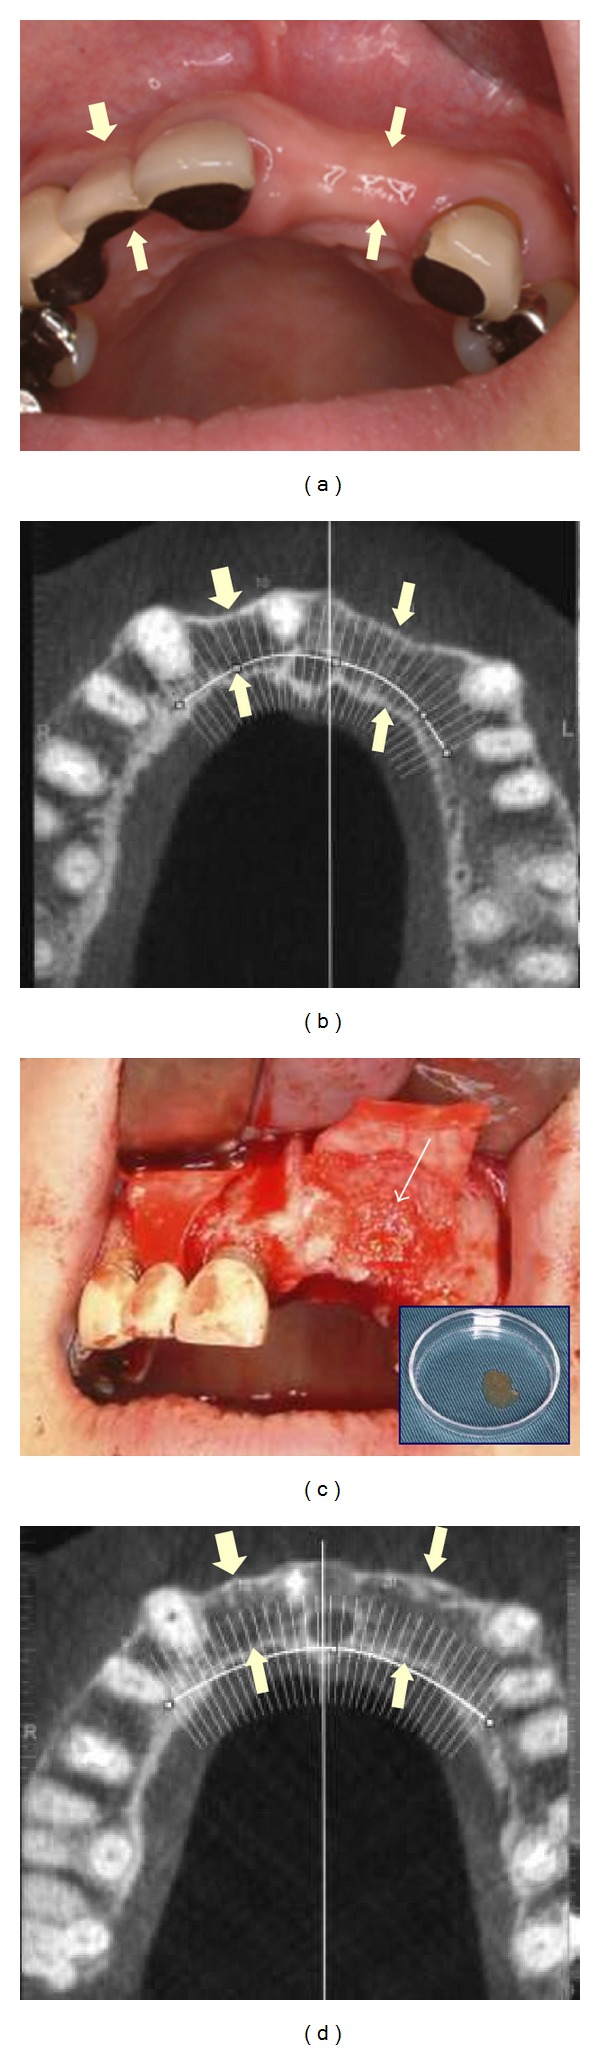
Alveolar ridge augmentation using bFGF-incorporated gelatin sponge and collagen membrane. (a) Preoperative intraoral photograph shows narrow alveolar ridge (arrows); (b) frontal plane of the preoperative dental CT is shown, and (c) bFGF-incorporated gelatin sponge (arrows) is implanted. Inserted photograph demonstrates bFGF-incorporated gelatin sponge, and (d) the narrow alveolar ridge is reconstructed (arrows) 8 months postoperatively.
